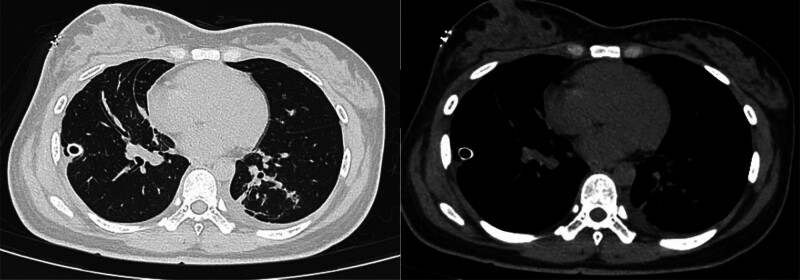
December 11, 2023 chest CT. CT = computed tomography.
